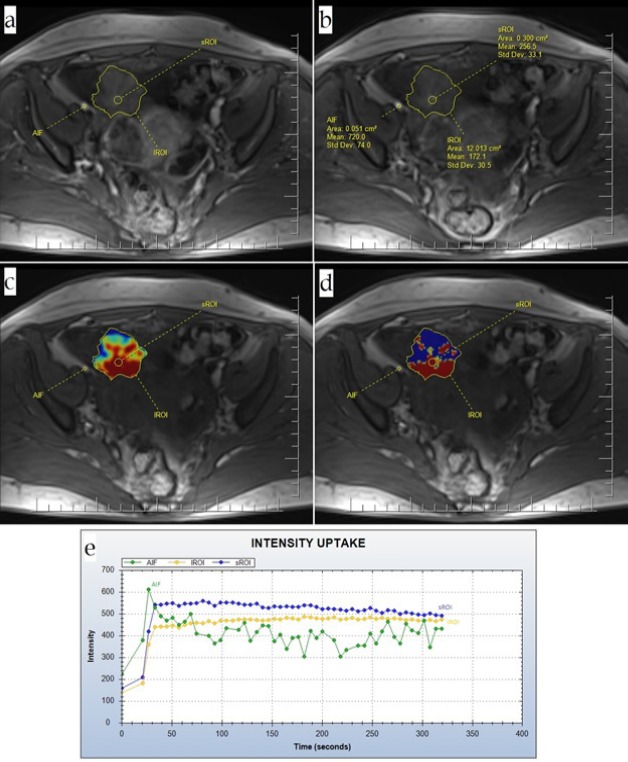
Type III Intensity Curve Along with the Corresponding Quantitative Measurements on the Patient’s MRI; (a) Source; (b) Details of the source; (c) Kep; (d) Ktrans; (e) TIC
